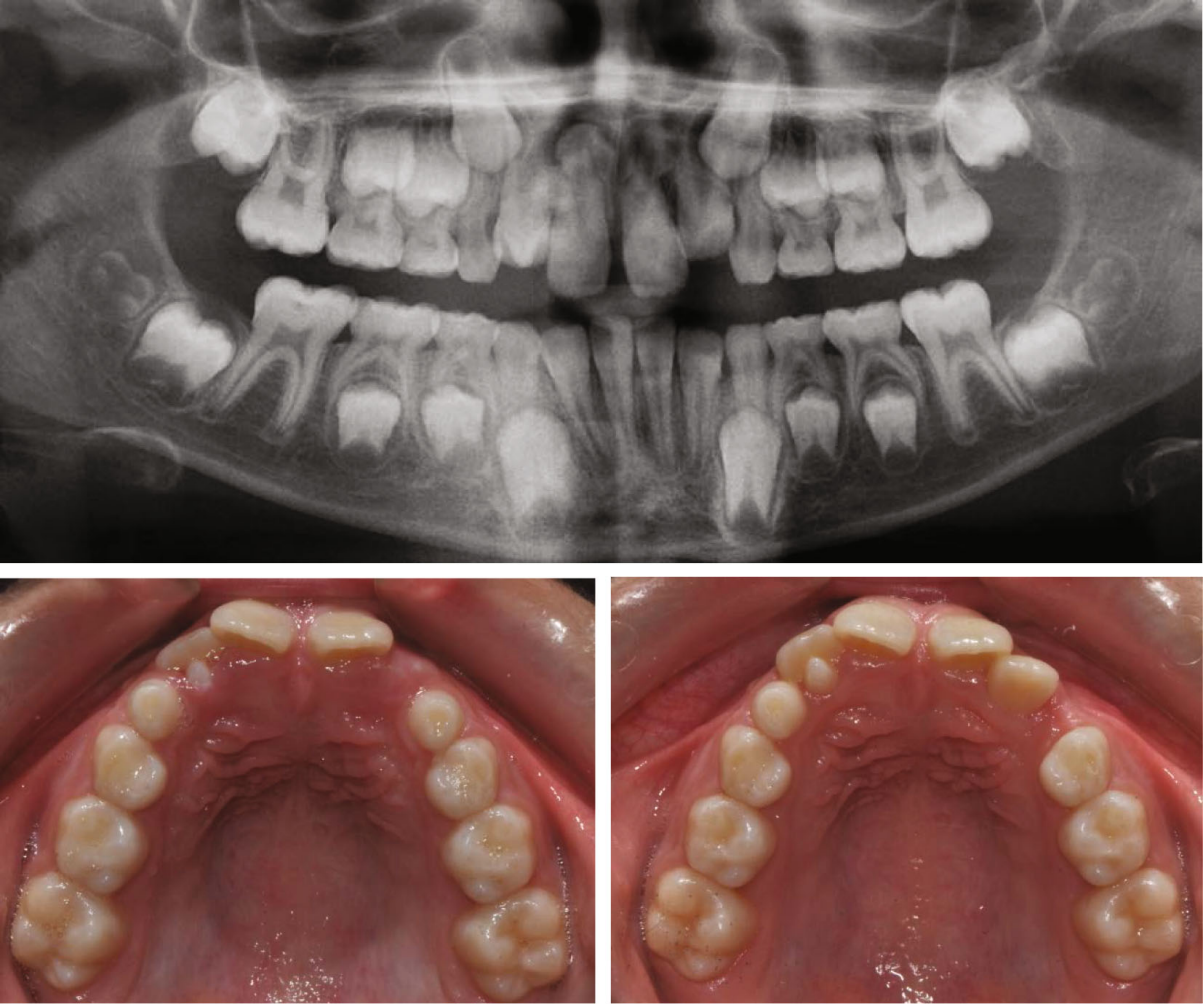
X-ray image and intraoral picture from the first clinical examination and 4 months later.
